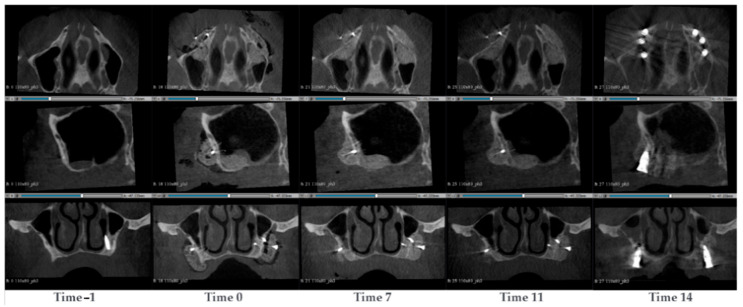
CBCT images of a 63-year-old man with atrophy in the maxillary sinus. SmartBone^®^ On-Demand plates were inserted in the left and right maxillary to recover the bone. The images show the progression of bone healing over time, from pre-surgery (Time −1) to 14 months post-surgery (Time 14).
